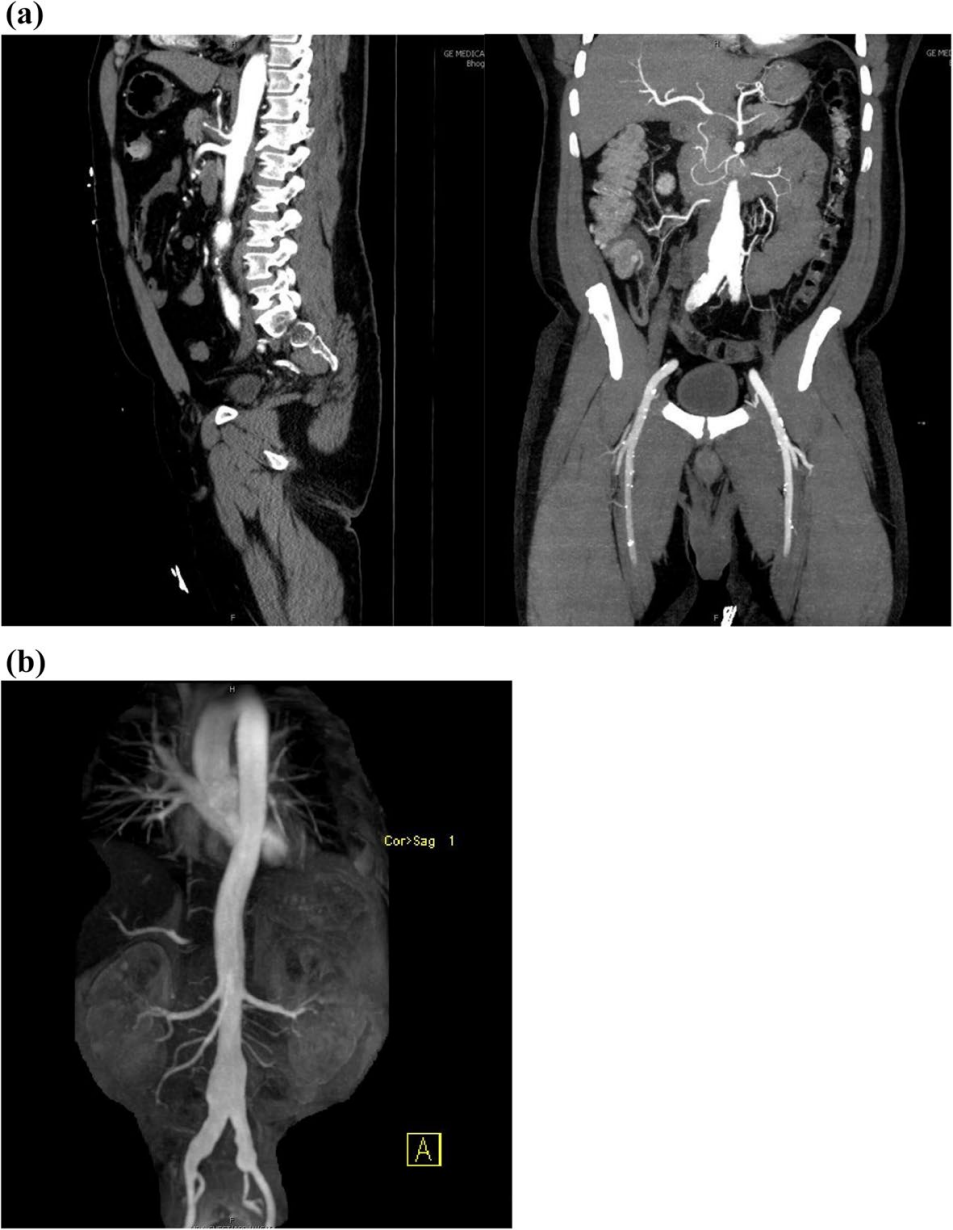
a CT Angiography – Abdominal Aortic Aneurysm, Celiac Trunk and Proximal branch narrowing (b) MRA – Abdominal Aortic Aneurysm and bilateral Femoral Artery Aneurysm
